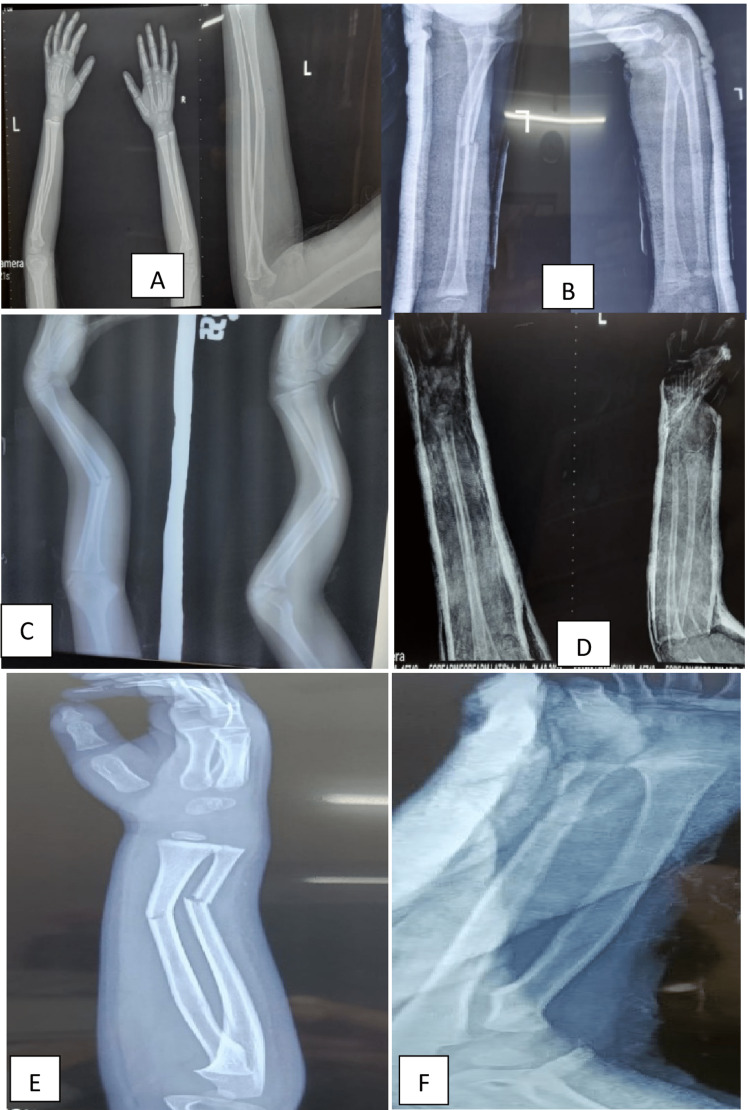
Pre-reduction and post-reduction X-rays of forearm fracture. (A) Pre-reduction X-ray showing greenstick fracture radius ulna. (B) Post-reduction X-ray. (C) Pre-reduction X-ray showing angulated fracture radius ulna. (D) Post-reduction X-ray. (E) Pre-reduction X-ray showing angulated fracture distal radius ulna. (F) Post-reduction X-ray.
